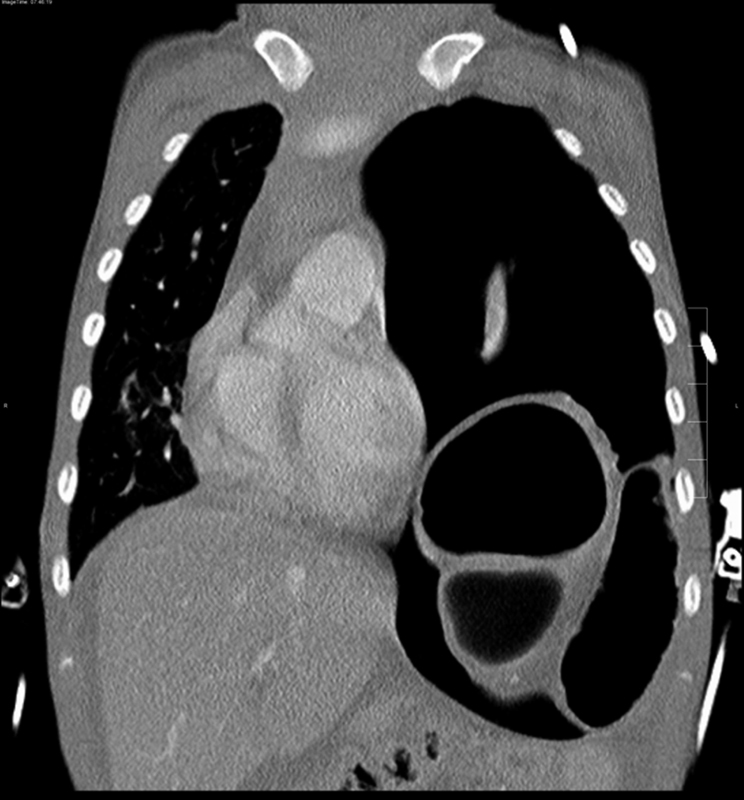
Thoracic CT scan on admission: left-sided tension pneumothorax with mediastinal shift. No further signs of a traumatic etiology.
